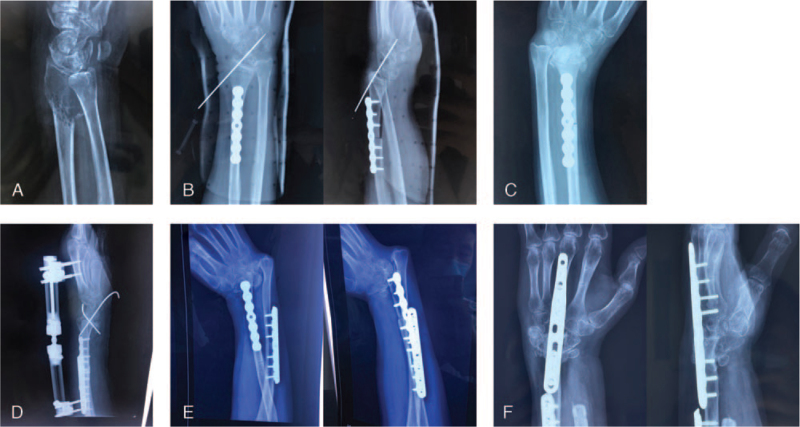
X-ray images of Case 1. A. Giant cell tumor of the left distal radius. B. Allogeneic bone transplantation. C. 5 months later, fractures and collapses of the distal radius and dislocation of the radiocarpal joint. D. 6 months after the first surgery, another surgery was performed. E. 22 months after the first surgery. F. Last follow-up, 15 months after ulna centralization.
